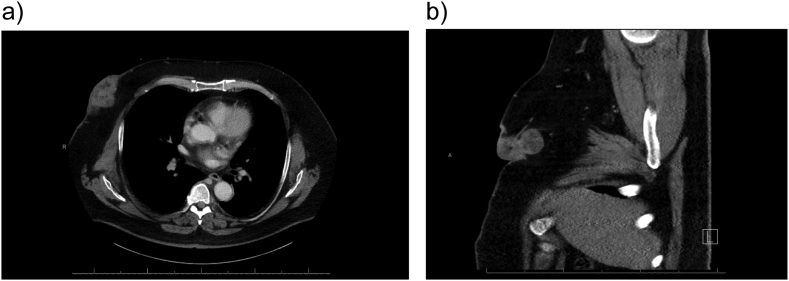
Computed tomography images showing the location and extension of the chest wall mass. a) axial cuts b) sagittal cuts.
